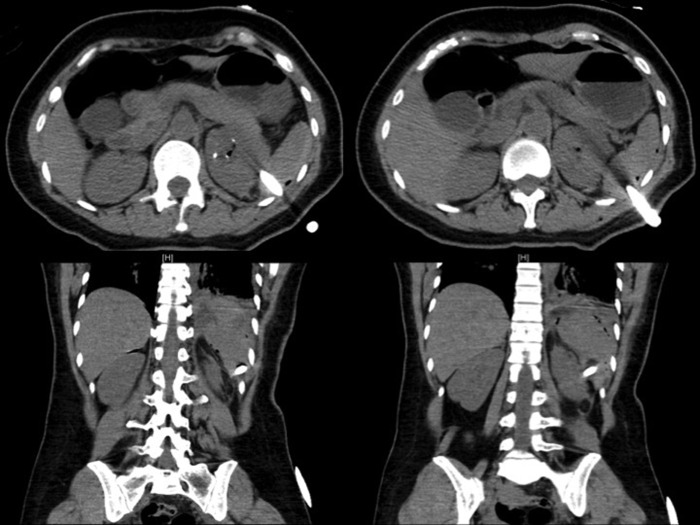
Axial and coronal computed tomography images showing trans-splenic nephrostomy catheter.
